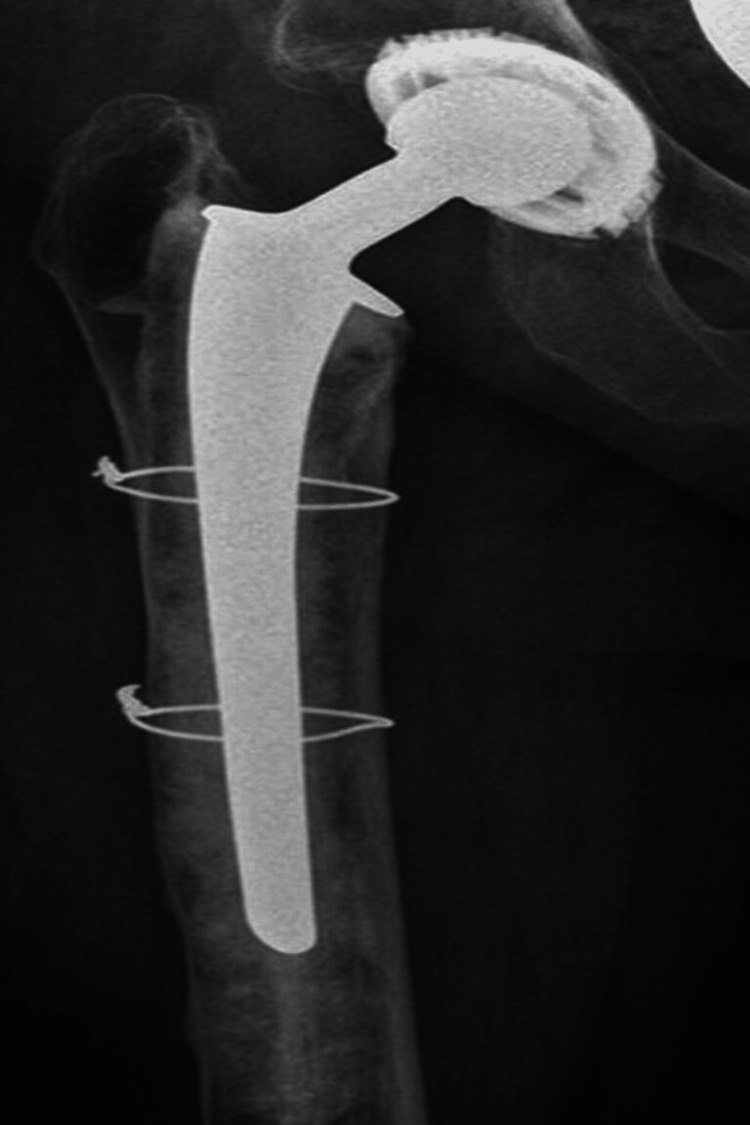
Cemented stem revision Follow-up radiograph two years after cemented stem revision with femoral impaction grafting. This image is reproduced with permission from Gehrke T, Gebauer M, Kendoff D. © 2013 British Editorial Society of Bone and Joint Surgery. All rights reserved [2].
